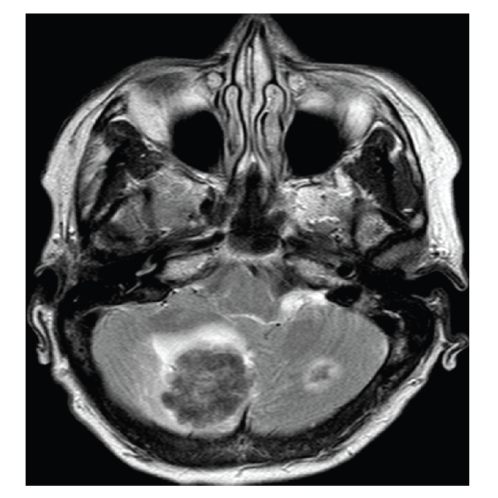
MRI of brain. Note space occupying lesions to cerebellum.
